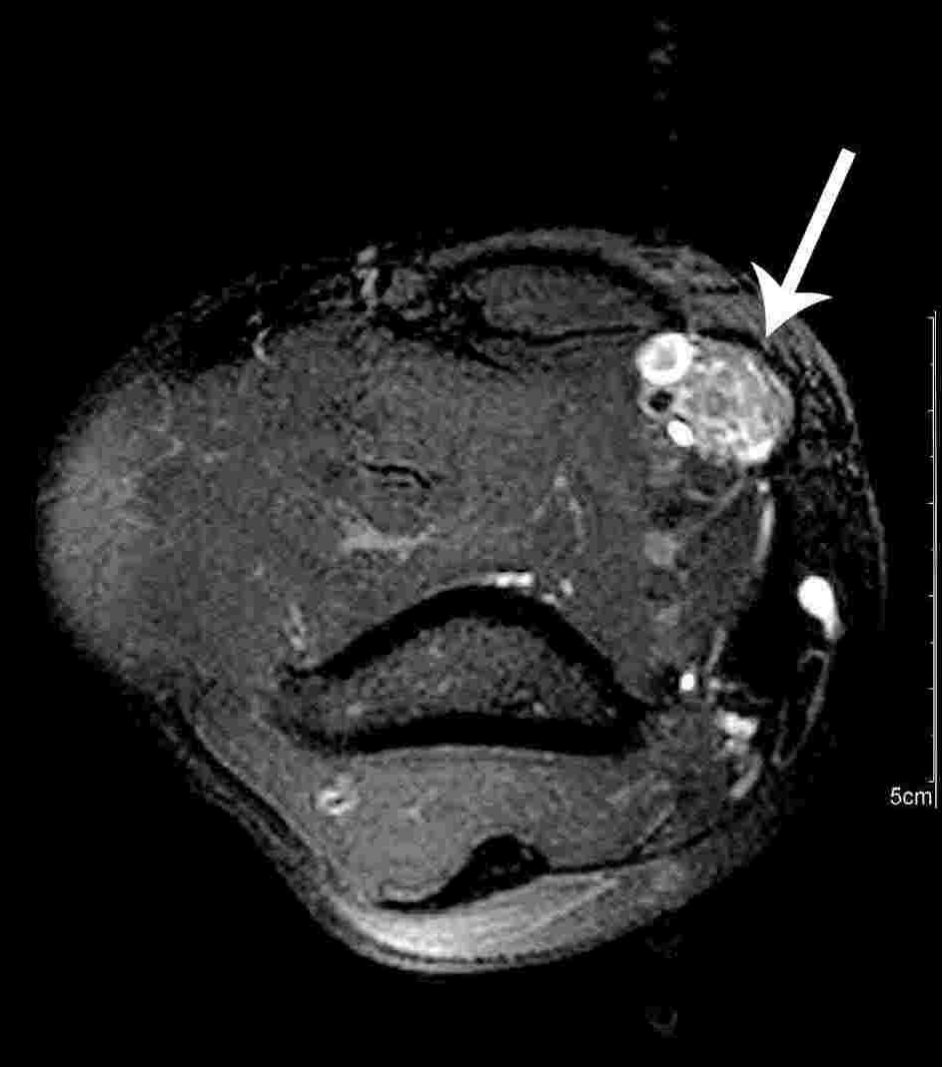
Axial fat-suppressed T2-weighted MRI shows a tumorous lesion of the median nerve with a fascicular pattern (arrow).
